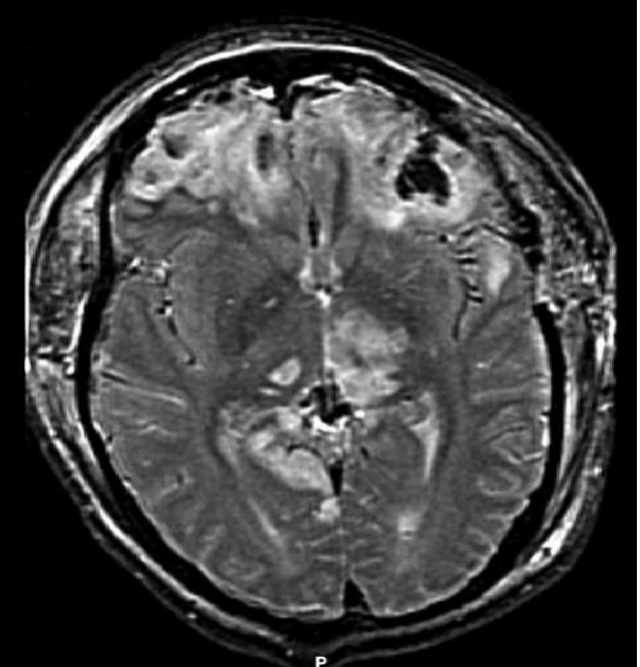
(MRI.tif) – Postoperative MRI showing evidence of injury due to herniation.
